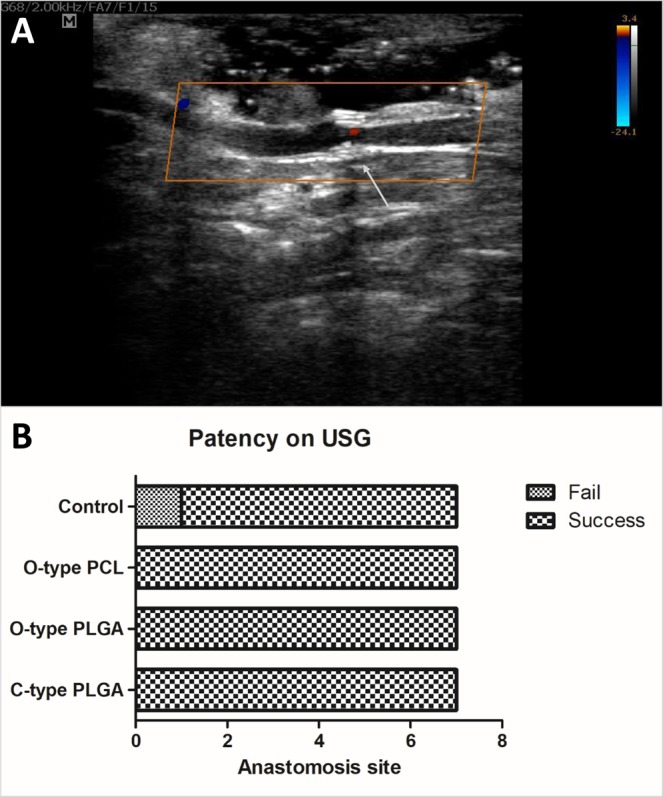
Ultrasonographic findings (USG) of anastomosed rabbit jugular veins. (A) The coupler is shown as hyperechoic echogenicity with posterior shadowing (arrow). Intraluminal flow was also detected by color duplex sonography. (B) Until 6 months, all coupler groups demonstrated intact intraluminal flow in ultrasonography. In one case in the control group, the author could not find an appropriate diameter of the jugular vein from 2 weeks, which was concluded to be a case of anastomotic failure.
